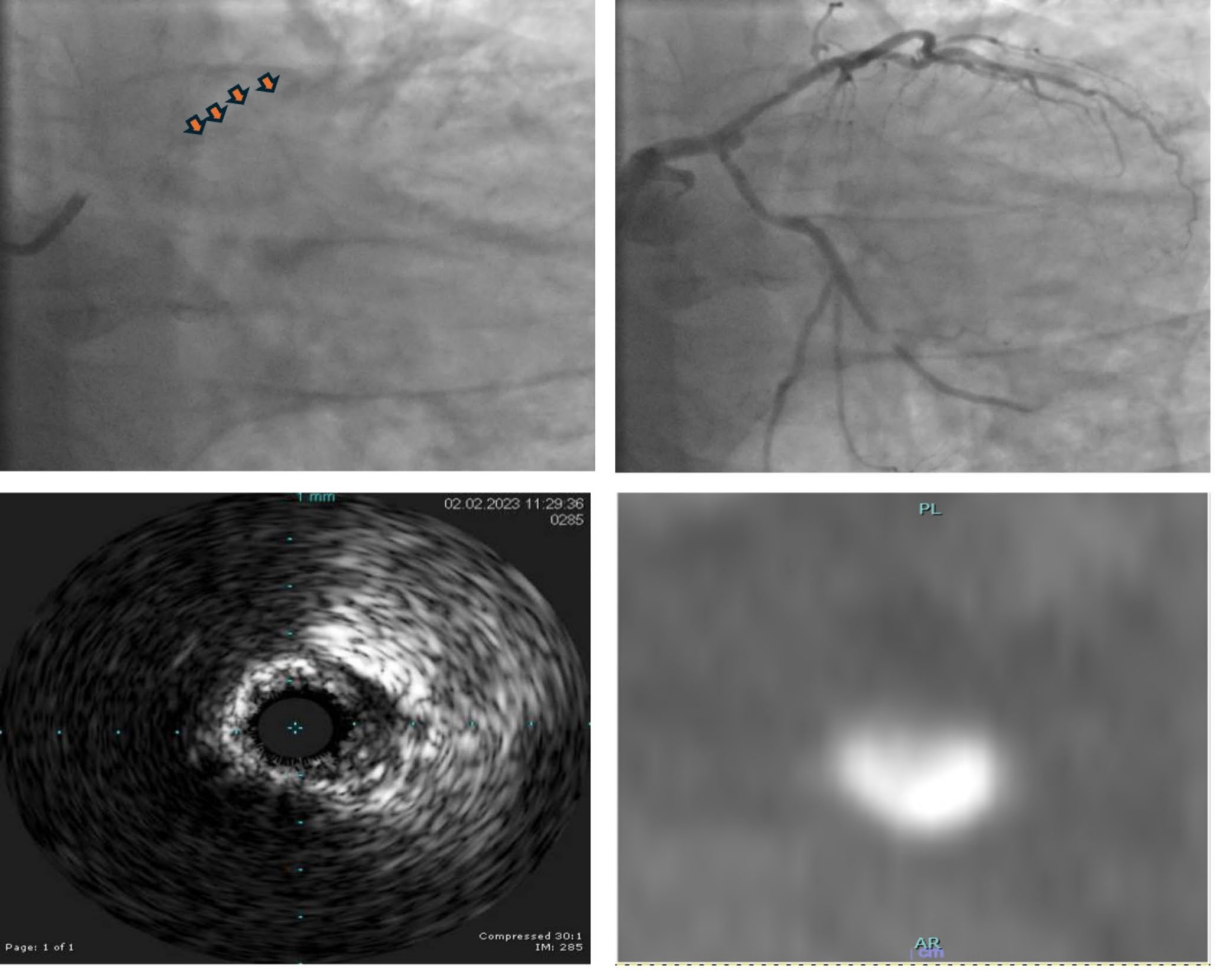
A representative example of a diagnostic angiogram pre-contrast injection with calcified CAD outlined with arrow heads (a), and post contrast injection with severe proximal LAD stenosis (b). Subsequent IVUS imaging with a 240° arc of coronary artery calcification (c). Cross-sectional CTCA imaging from the same location with 240° of calcification and calcium density of 1619 HU (d). CAD-coronary artery disease. LAD = left anterior descending.IVUS = intravascular ultrasound imaging. CTCA = computed tomography coronary angiogram.HU = Hounsfield units
